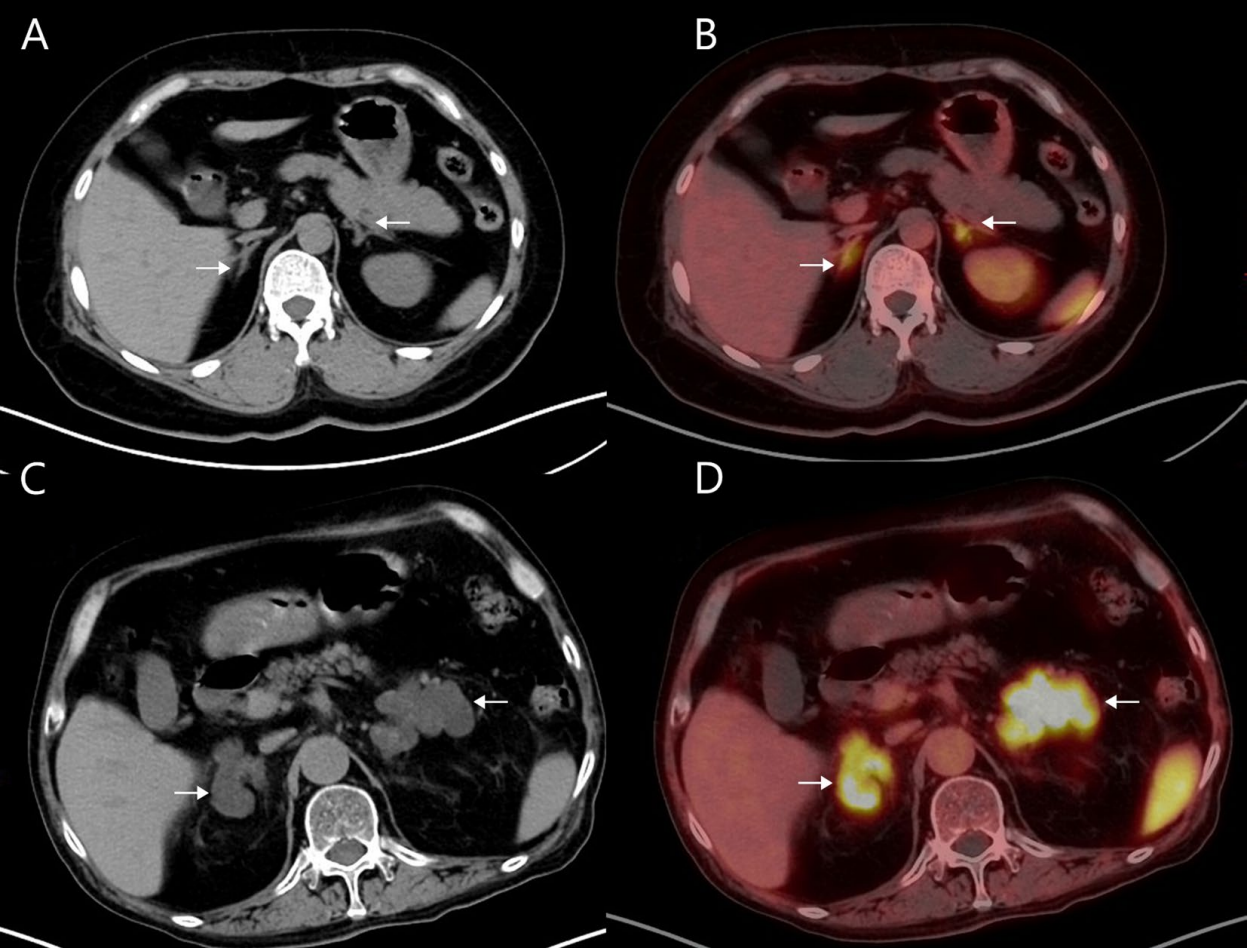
CXCR4-PET/CT images from one patient with Cushing’s disease caused by a pituitary tumor and one patient with adrenal macronodular hyperplasia. (A-B) Cushing’s disease (pituitary tumor). The patient exhibited no Cushingoid facies, with a BP of 118/75 mmHg, cortisol level of 530.8 nmol/L, and ACTH level of 72.9 ng/L. PET imaging revealed mild, uneven thickening of both adrenal glands, with a maximum diameter of 7 mm. On the left side, SUVmax was 4.9, and SUVavg was 3.1. On the right side, SUVmax was 4.8, and SUVavg was 2.9, with no significant asymmetry observed. (C-D) Cushing’s syndrome. The patient presented with central obesity, a full moon face, and a BP of 172/102 mmHg. Cortisol levels were 1468.2 nmol/L, and ACTH levels were 6.3 ng/L. The left adrenal gland exhibited a large lesion, measuring 56 × 40 mm, with an SUVmax of 13.4 and an SUVavg of 8.5. The right adrenal lesion measured 39 × 38 mm, with an SUVmax of 10.3 and an SUVavg of 6.6. The left adrenal gland was more dominant, leading to a subsequent left adrenalectomy. Following surgery, BP decreased but did not return to normal
